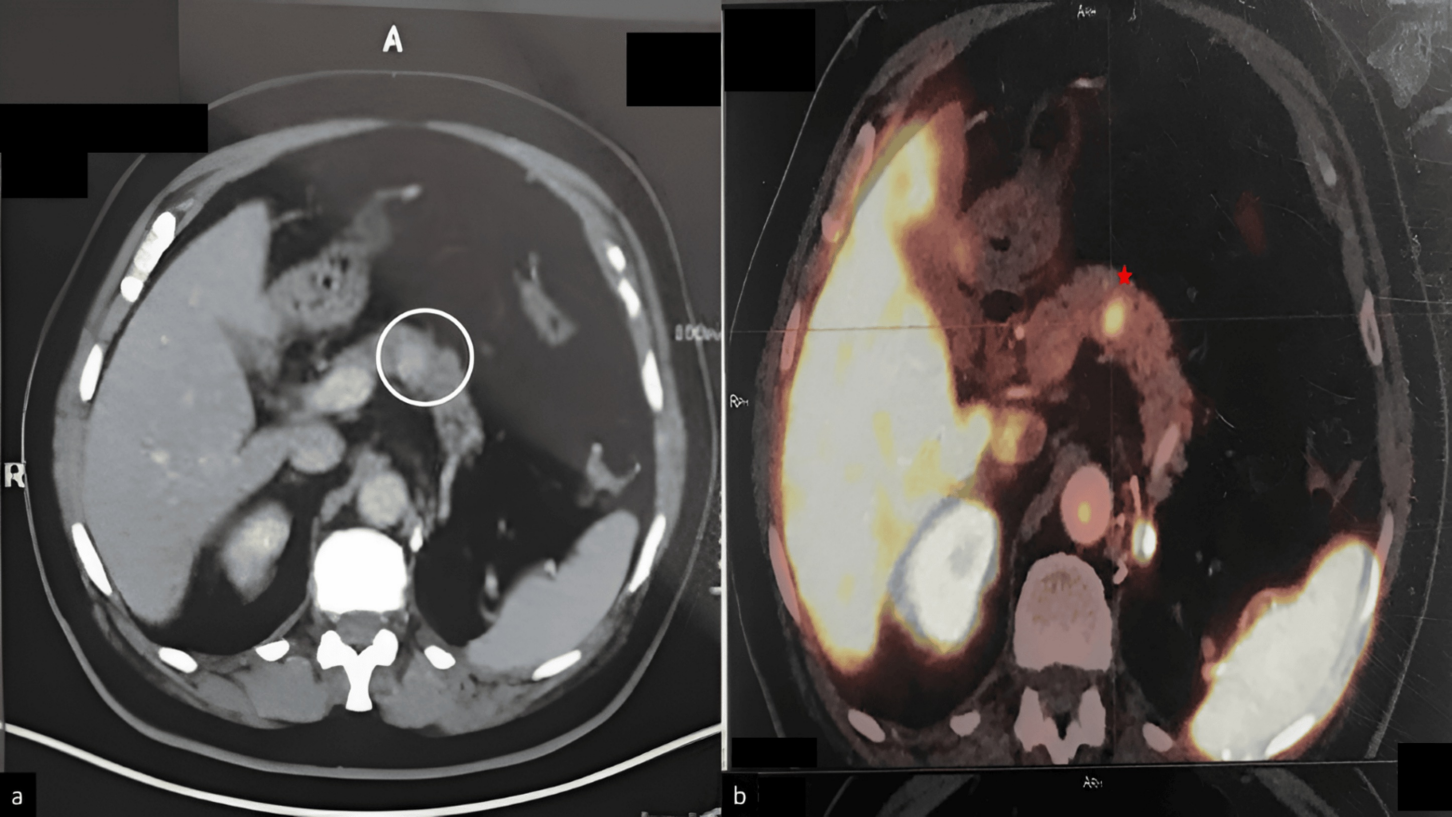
(a) Contrast-enhanced CT of the abdomen showing a hyperenhancing area measuring 18 × 15 mm in the body of the pancreas (encircled). (b) PET scan displaying focal uptake in the proximal body of the pancreas* and no evidence of residual disease at the postoperative site (left iliac fossa).
